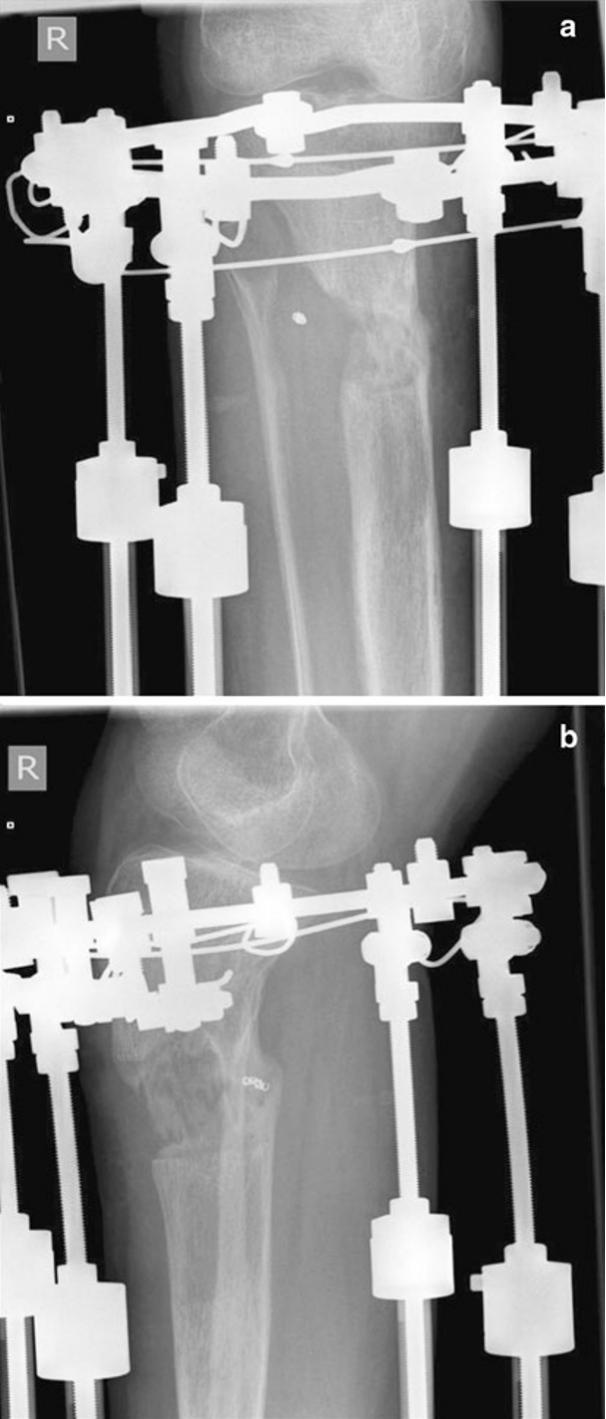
a Anteroposterior and b lateral radiograph of the tibia 1-month after angiographic coil embolisation of the pseudoaneurysm arising from the anterior tibial artery. A reduction in the concavity of the regenerate was noted. The embolisation coil can be seen at the site of the pseudoaneurysm
